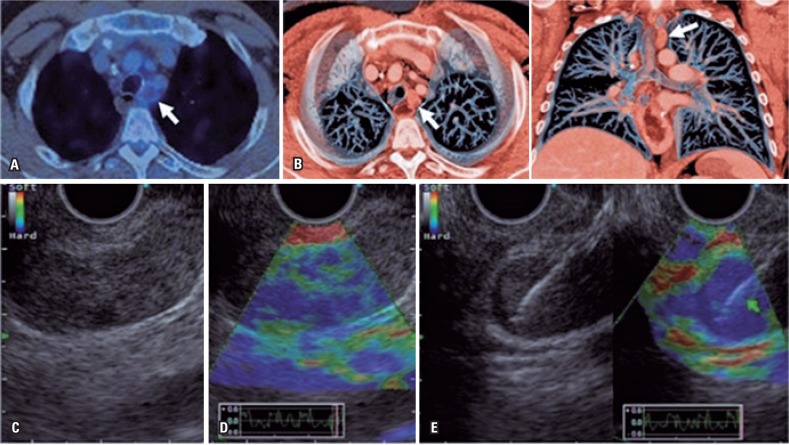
Paratracheal lymph node. (A) positron tomography emission imaging with left paratracheal lymph node (SUV 5.5); (B) Reconstruction in chest tomography in axial and coronal cuts, with increased lymph nodes (white arrow); (C) Endoscopic ultrasound showed hypoechogenic, homogenous lymph node measuring around 25mm; (D) Qualitative elastography with intermediary rigidity characteristics (blue and green); (E) Echoguided puncture
